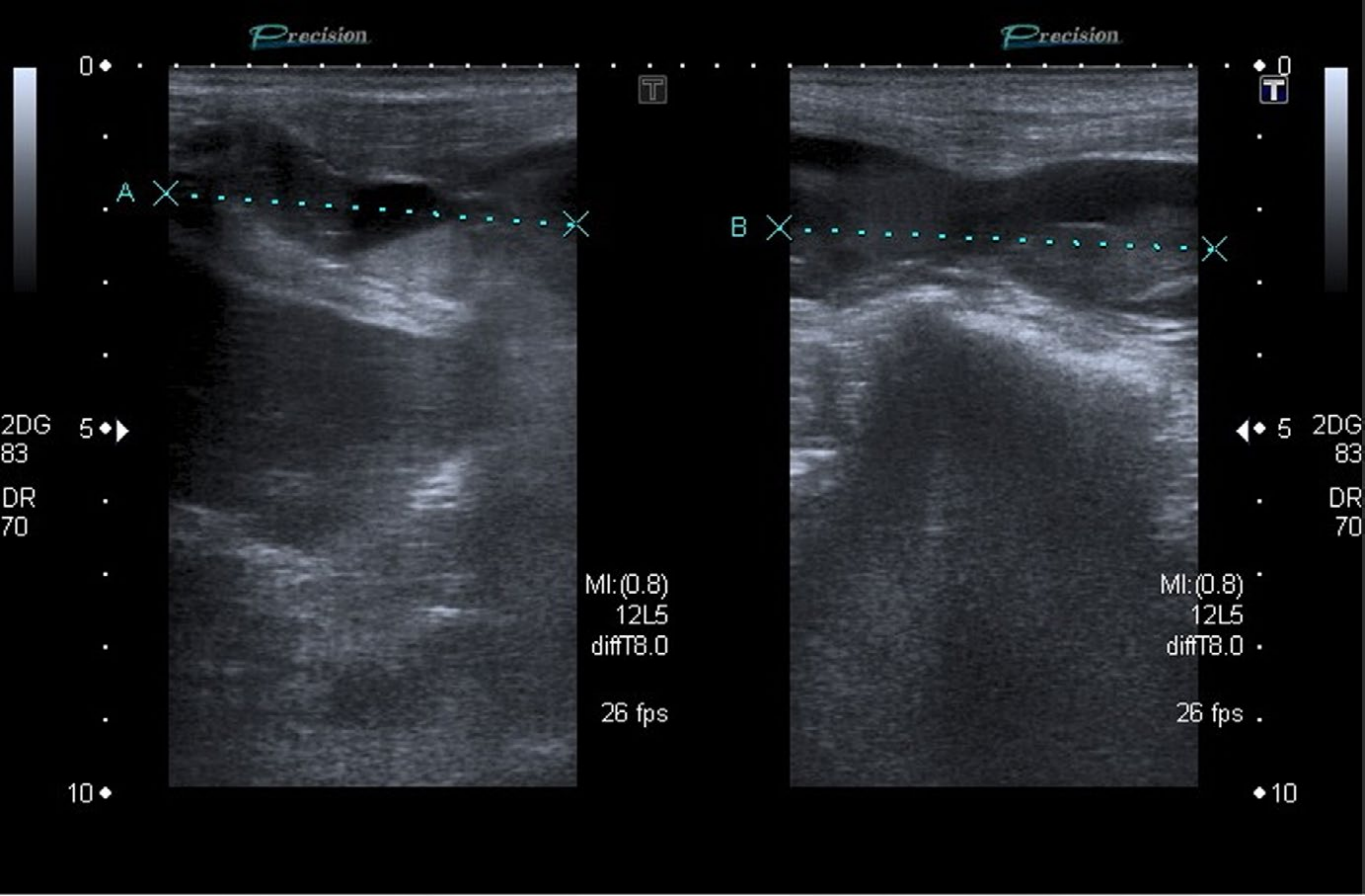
Case 2: Ultrasound of the abdomen revealing a right‐sided rectus sheath hematoma. Diffuse wall thickening from the upper to the lower insertion of the rectus abdominis muscle, with several internal liquid loci, within which solid material is observed, outlining the solid/liquid level, which is compatible with a hematoma (probably spontaneous) in the ultrasound image
